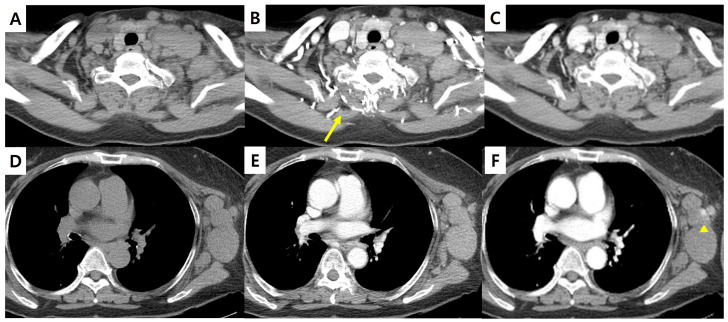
Chest CT images of a 64-year-old woman with lymphoma. (A,D) NCCT, (B,E) CECT, and (C,F) sCECT. Flow artifact from collateral vessel contrast filling (arrow) is prominent on CECT but markedly reduced on sCECT. Mild over-enhancement of non-specific areas, such as the left axilla (arrowhead), was occasionally observed. CECT = contrast-enhanced CT; NCCT = non-contrast CT; sCECT = synthetic contrast-enhanced CT.
